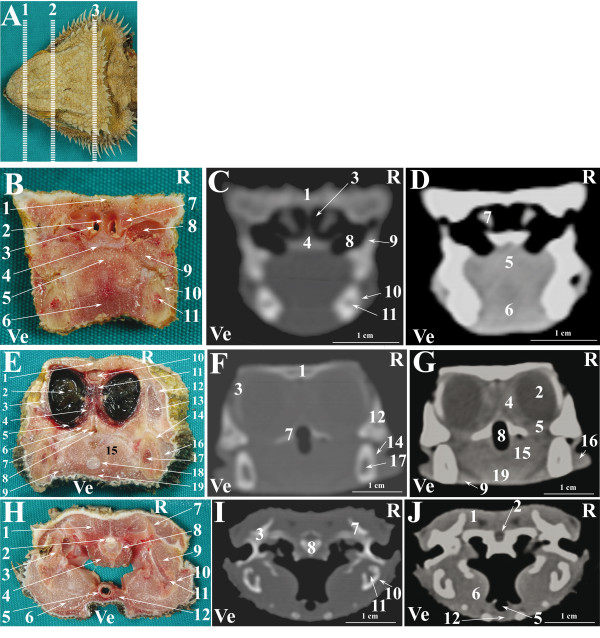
Normal computed tomographic anatomy of the head of the bearded dragon.A. Photograph of the head of a bearded dragon; lines (1–3) indicate the approximate levels of matched cross-sections and CT images. B- J: Matched images of cross-sectional gross anatomy ( B- E- H), CT image displayed in bone window (WW: +2000; WL: +500)(C-F-I) and CT image displayed in soft tissue window (WW: +400; WL: +40) ( D- G- J) corresponding to: line 1 ( B- C- D), line 2 ( E- F- G) and line 3 ( H- I- J) as depicted in Figure 9A. R is right and Ve is ventral. B- C- D: 1. Nasal bone; 2. Nasal septum; 3. Choanae; 4. Vomer bone; 5. Tongue; 6. Musculus intermandibularis + Musculus geniohyoideus + Musculus genioglossus; 7. Nasal glands; 8. Choanal tube; 9. Maxillary bone; 10. Dentary bone; 11. Meckelian canal. E- F- G: 1. Frontal bone; 2. Eye; 3. Scleral ossicles; 4. Harderian gland; 5. Sinus orbitalis; 6. Palatine gland; 7. Pterygoid bone; 8. Cavum oris; 9. Sublingual gland; 10. Brain; 11. Choroid plexus; 12; Postorbital bone + Jugal bone; 13. Musculus adductor mandibulae externus superficialis; 14. Dentary bone; 15. Tongue; 16. Infralabial glands; 17. Meckelian canal; 18. Entoglossal process of the hyobranchial skeleton; 19. Musculus intermandibularis + Musculus geniohyoideus + Musculus hyoglossus + Musculus pterygoideus typicus. H- I- J: 1. Musculus adductor mandibulae externus medialis; 2. Brain; 3. Quadrate bone; 4. Musculus longus colli; 5. Trachea; 6. Musculus pterygoideus typicus; 7. Postparietal process of otooccipital bone; 8. Orbitosphenoid bone; 9. Musculus adductor mandibularis externus superficialis; 10. Angular bone + Surangular bone; 11. Adductor fossa; 12. Musculus intermandibularis + Musculus geniohyoideus + Musculus ceratohyoideus + Musculus hyoglossus.
